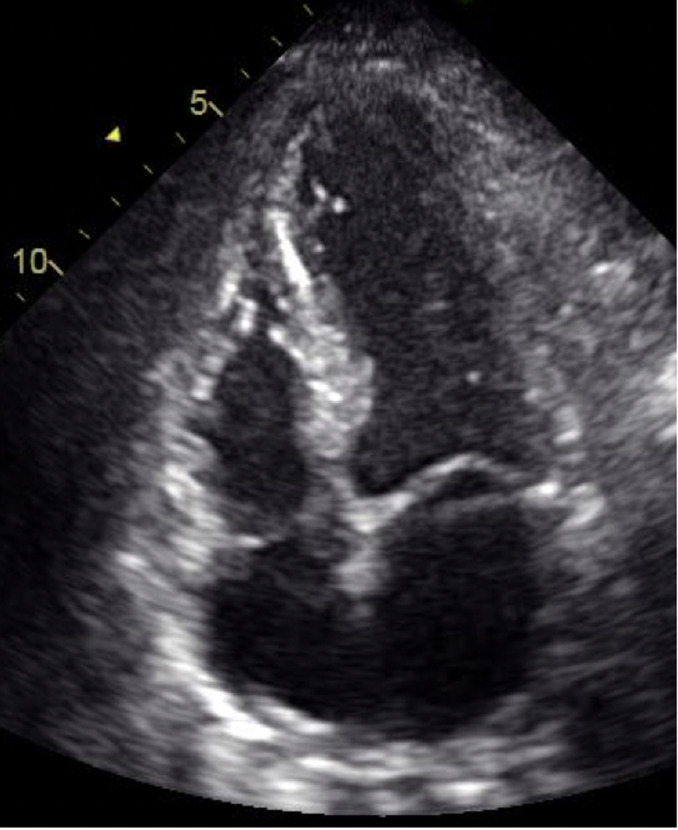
end-systolic frame of apical 4-chamber demonstrating features of apical ballooning suggestive of Takotsubo cardiomyopathy
